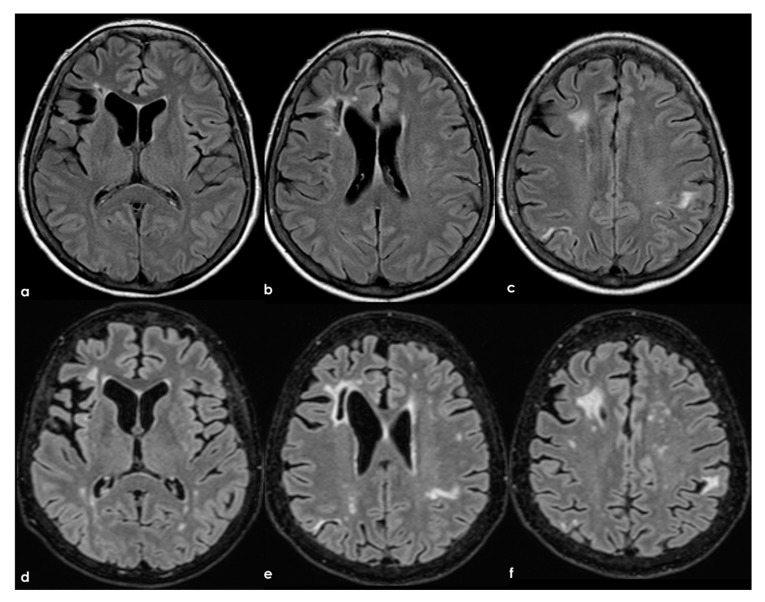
Brain MRI (axial FLAIR) at baseline (panels (a–c)) and after 8 years (panels (d-f)), showing the increase of WMHs in the subcortical white matter.
